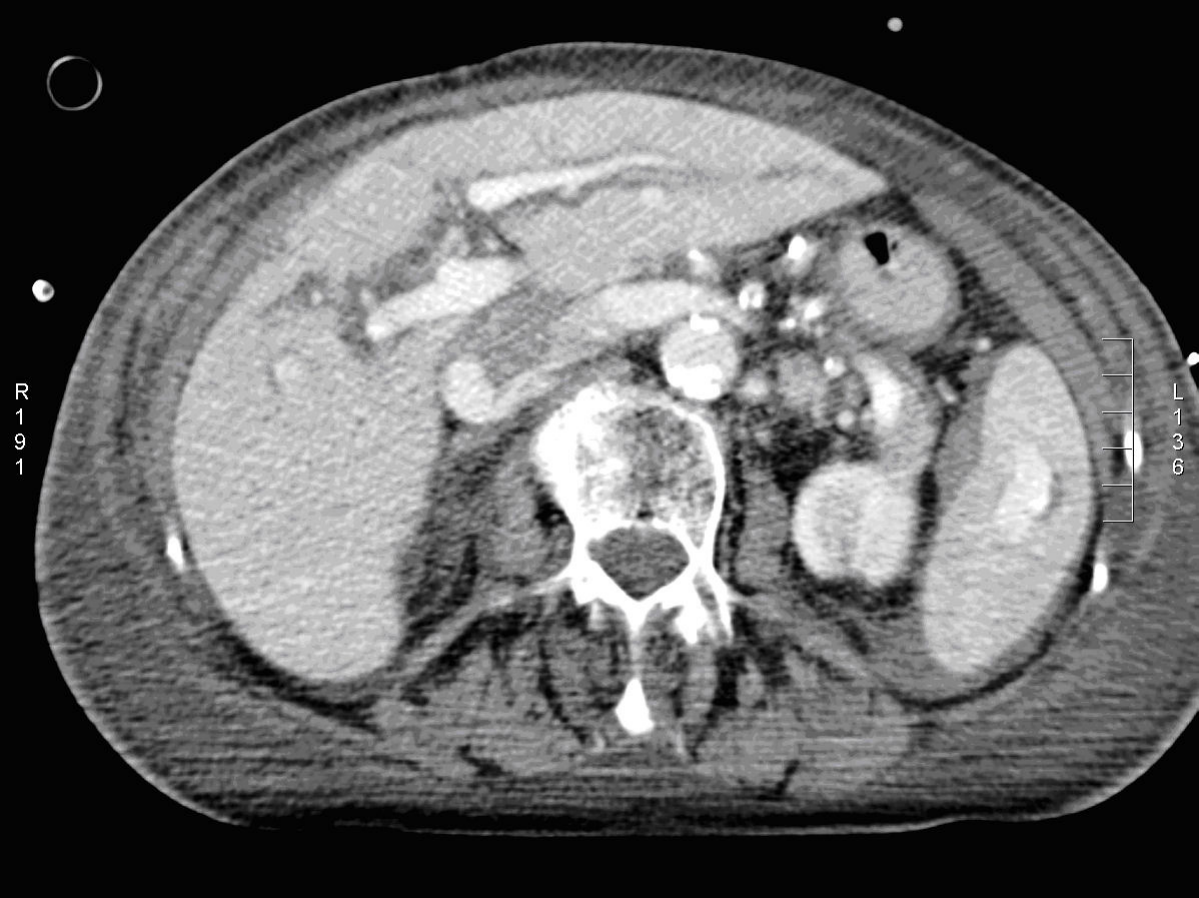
Computed tomography with intravenous contrast shows small amounts of hemoperitoneum around the spleen and a contrast 'blush' confined to the splenic parenchyma.
